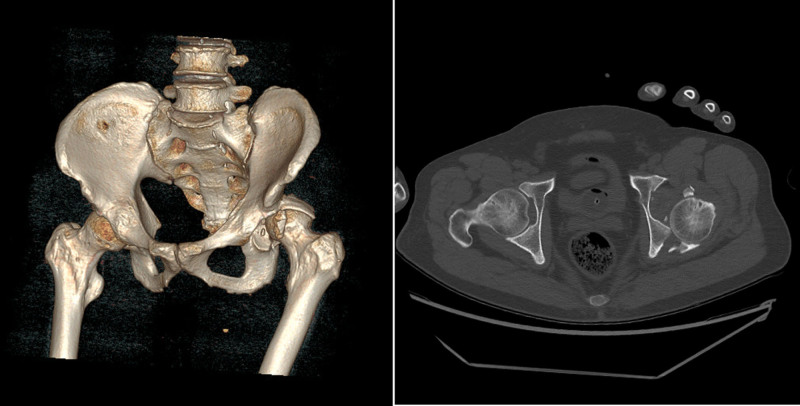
A 3D-reconstructed acetabular fracture visualized by pelvic computed tomography (CT) imaging. CT = computed tomography.
